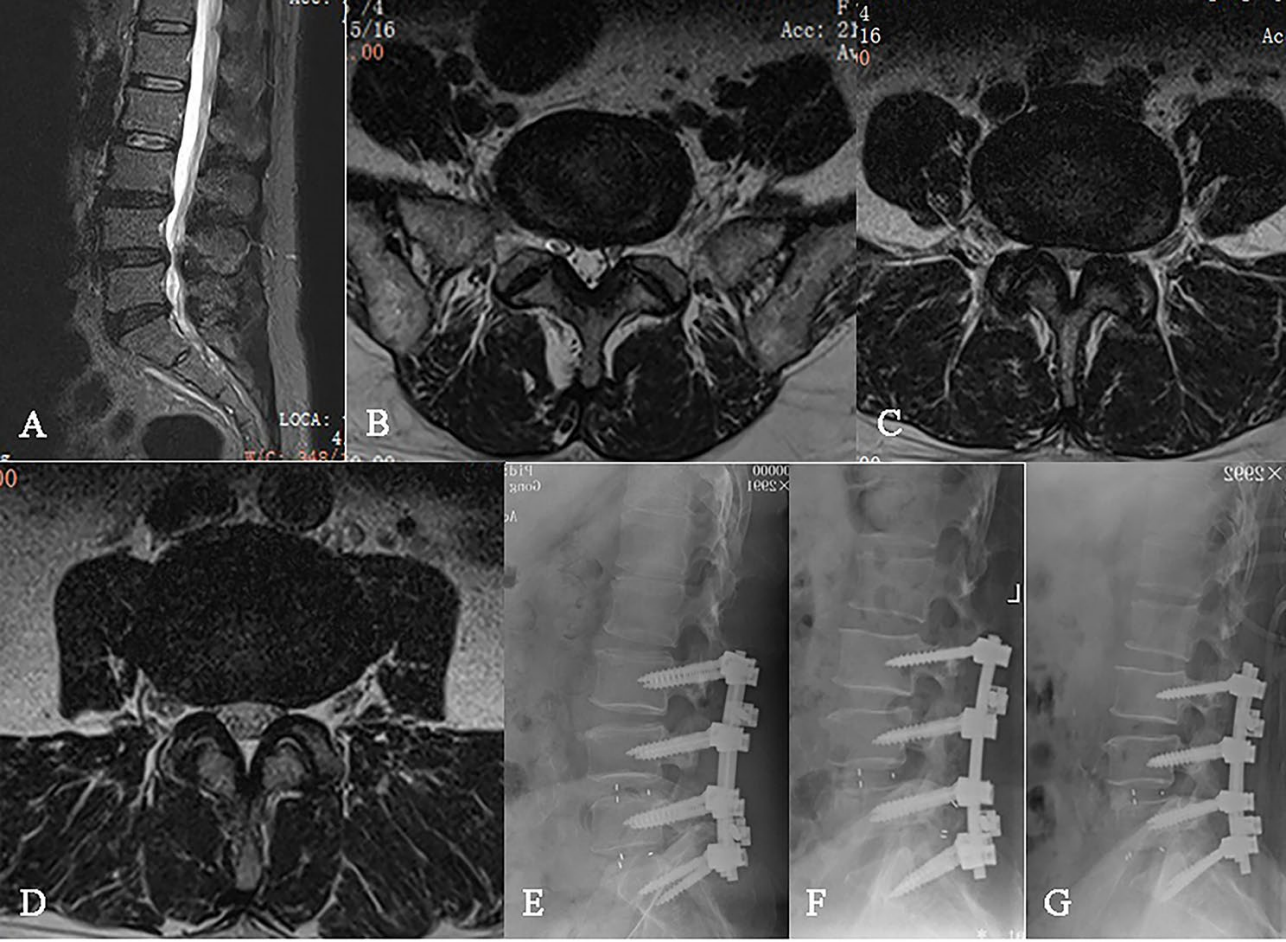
Preoperative lateral MRI of a 62-year-old female with lumbar spinal stenosis (A); MRI of the patient at L5/S1 (B), L4/5 (C), and L3/4 (D) levels; Radiographic view of the patient at 3rd day (E), 1st month (F) after first operation and after second operation (G)
